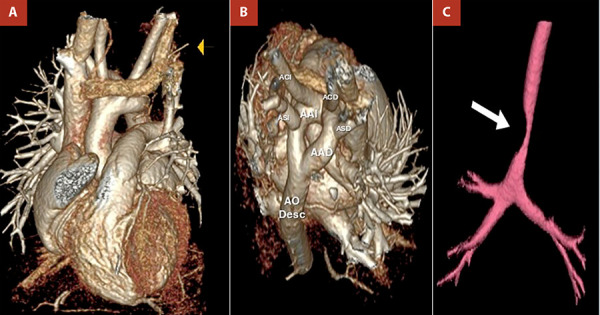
Three-dimensional (3D) reconstruction of chest CT angiography. (A) Anterior view showing the origin of the right and left aortic arches from the ascending aorta. (B) Right posterosuperior view demonstrating the aortic arches forming a complete vascular ring. (C) Severe extrinsic compression of the distal trachea. RAA: right aortic arch. LAA: left aortic arch. RCCA: right common carotid artery. LCCA: left common carotid artery. RSA: right subclavian artery. LSA: left subclavian artery. Desc. Ao.: descending aorta.
